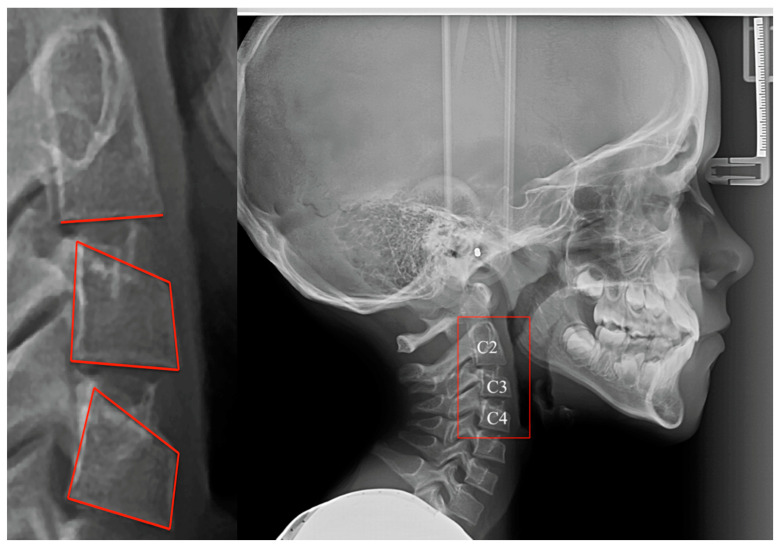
Lateral cephalometric radiograph with the 2, 3, and 4 cervical vertebrae highlighted.
